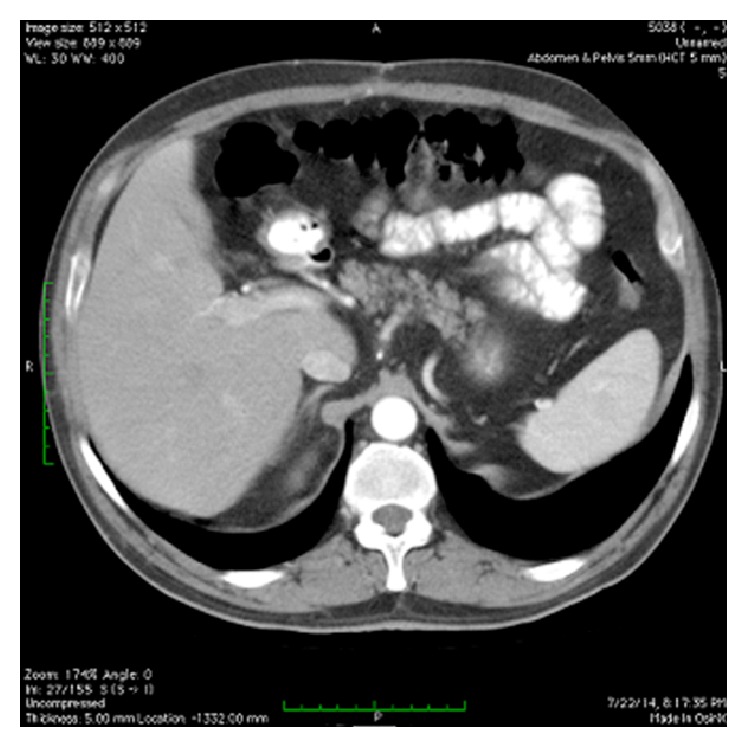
Postoperative CT of the same patient.
